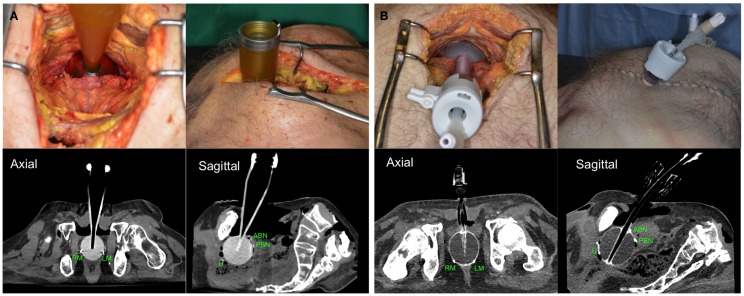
Positioning of Zeiss Intrabeam™(A) or Xoft Axxent eBx™[(B) through laparoscopic trocar] applicators and CT scans of prostatectomized corpses with radiopaque clips located at different target tissues. U, urethra; ABN, anterior bladder neck; PBN, posterior bladder neck; LA, left apex; RA, right apex.
